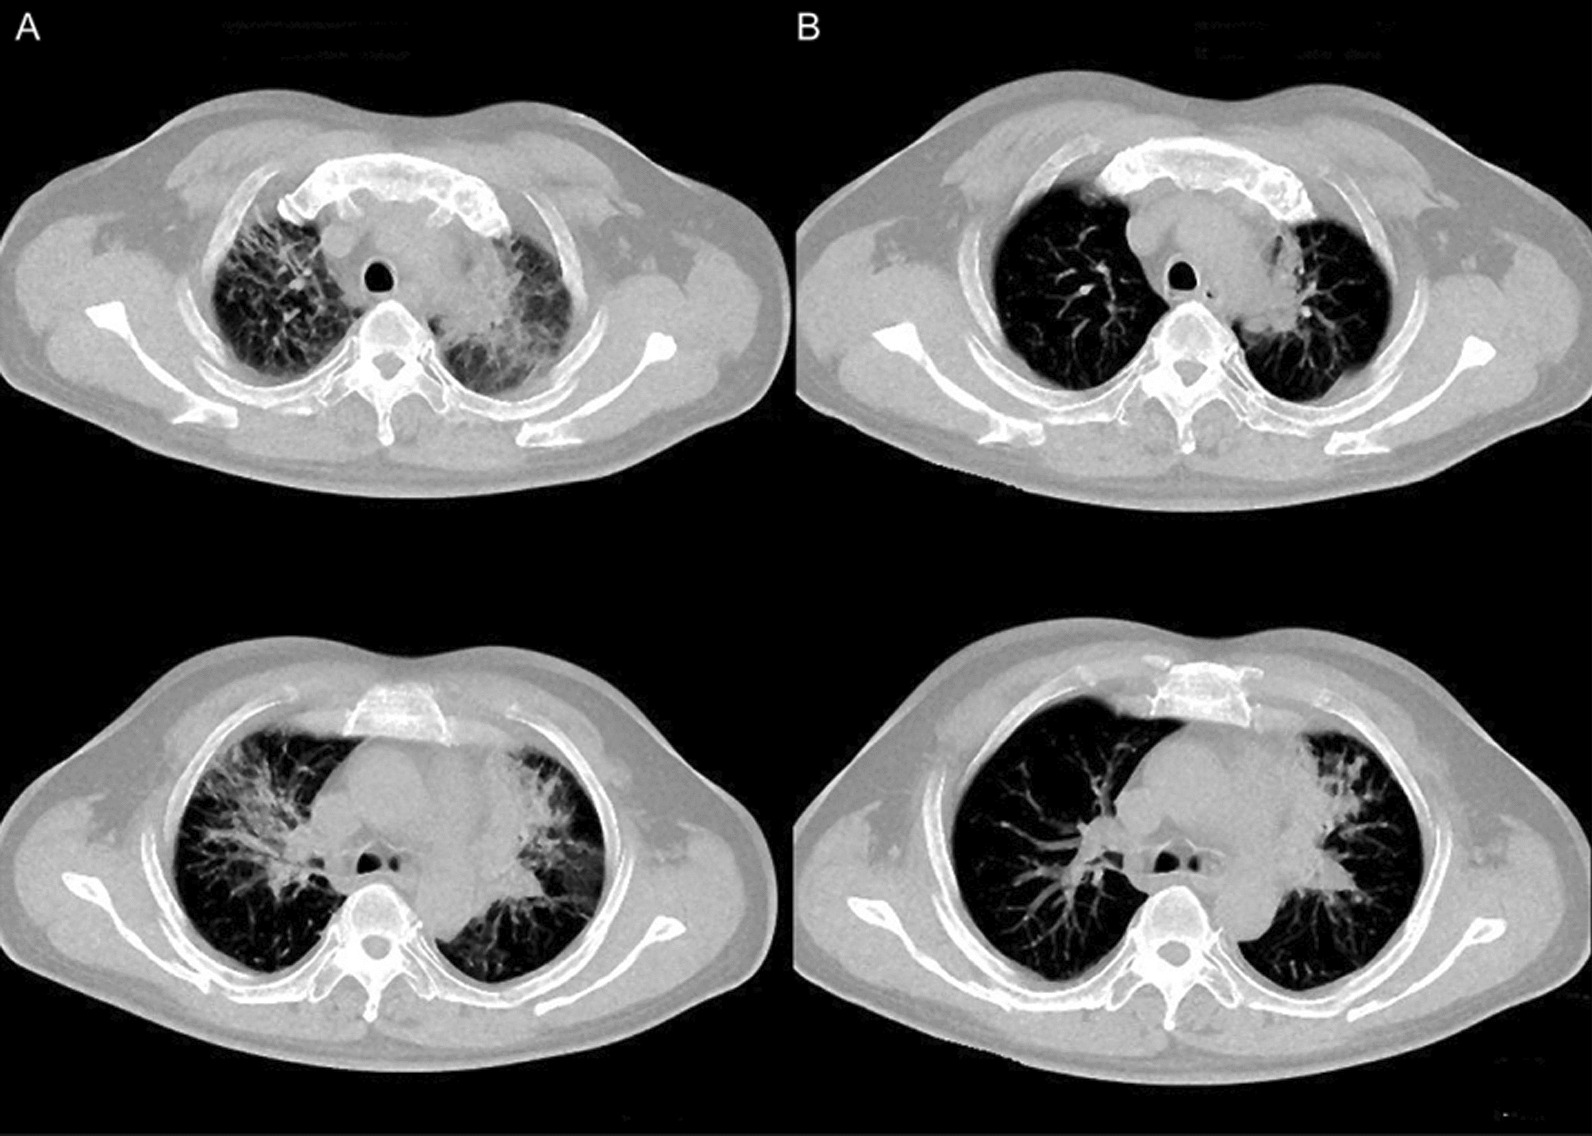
The chest CT scan demonstrated pneumonia of Brigatinib. A The Brigatinib treatment induced interstitial pneumonia. B The treatment with methylprednisolone was performed and pneumonia was resolved
